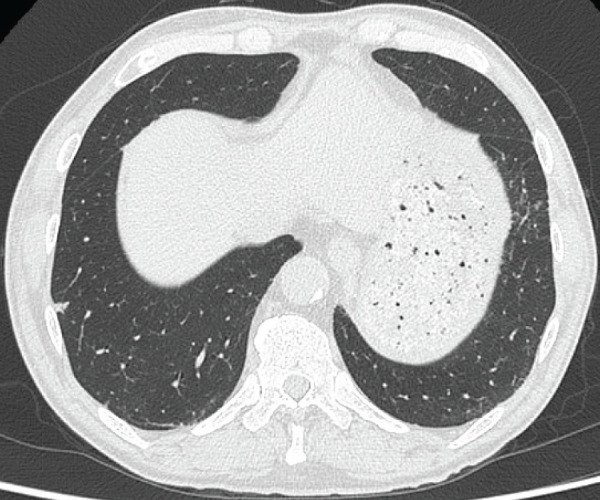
Chest CT showing an 8-mm solid nodule in the peripheral segment 8 (S8) of the right lower lobe (Case 1).
